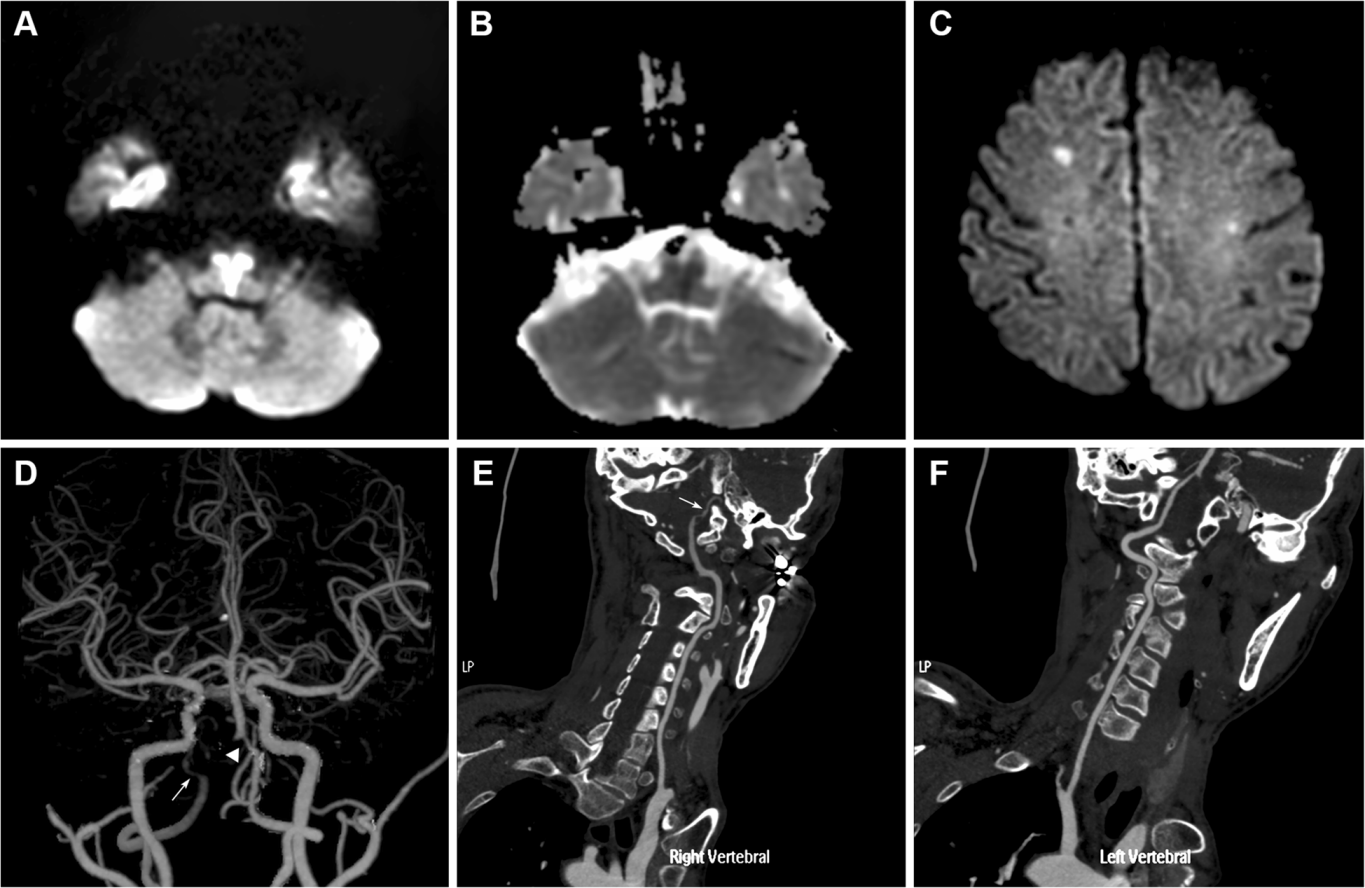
a Brain diffusion-weighted image on admission showing a “heart-shaped” hyperintense area in the bilateral ventral medulla, characteristic of bilateral MMI. b Corresponding apparent diffusion coefficient map, showing restricted diffusion within the heart-shaped lesion. c Diffusion-weighted image at 40 days after admission showing two small subcortical infarcts in bilateral cerebral hemisphere. d-f Computed tomography angiogram showing mild stenosis in the left vertebrobasilar junction (arrowhead) and anatomic variability of the right vertebral artery, which extended directly into the posterior inferior cerebellar artery (arrows)
